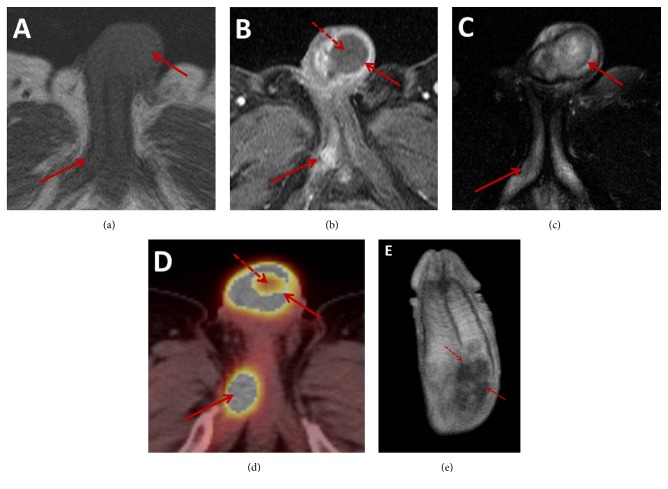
Axial T1-weighted MR images (a) demonstrate two isointense penile masses involving the left paracentral shaft base and proximal right corpus cavernosum (solid arrows) demonstrated with enhancement (solid arrow) on axial contrast enhanced T1-weighted MR images (b). The larger lesion at the base of the shaft demonstrates peripheral enhancement and central nonenhancement (dashed arrow), while the smaller posterior lesion homogeneously enhances. Both lesions demonstrate intrinsic heterogeneous hyperintensity (solid arrow) on axial T2-weighted MR imaging (c). Corresponding to the areas of enhancement on postcontrast MR images, PET/CT imaging (d) demonstrates hypermetabolic activity (solid arrow). A central area of decreased metabolic activity within the larger lesion (dashed arrow) corresponds to the area of central nonenhancement on MRI in keeping with central necrosis. Coronal contrast-enhanced T1-weighted MR image (e) demonstrates the larger lesion (solid arrow) extending across the intercorporal septum (dashed arrow).
